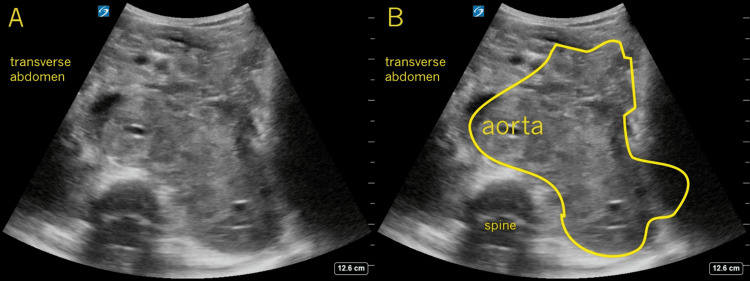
Transverse Mid-abdomen In this transverse image taken from the mid-abdomen, landmarks such as the transverse spine (frame B labeled) and the aorta (frame B labeled) can help orient the operator. A large, slightly irregular, mostly echogenic mass is identified that displaces and distorts the normal abdominal structures. Again, an operator with a basic understanding of normal sonographic anatomy should recognize the abnormal appearance of these findings.
